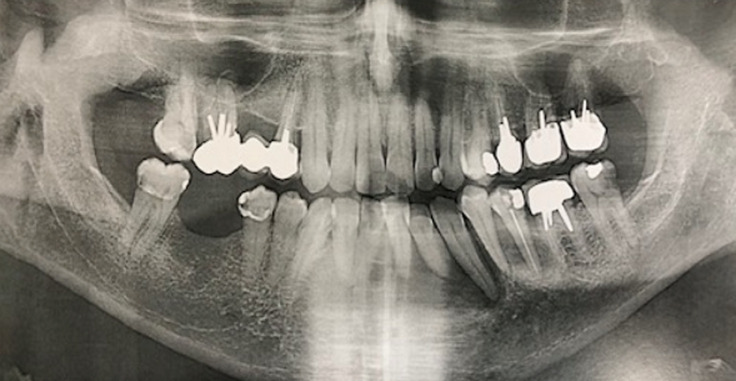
panoramic X-ray: radiolucent mandibular opposite teeth #35 to 42
